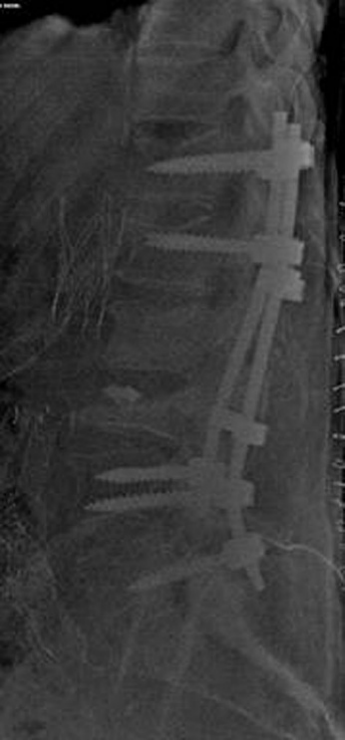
Postoperative X-ray of lumbar spine
